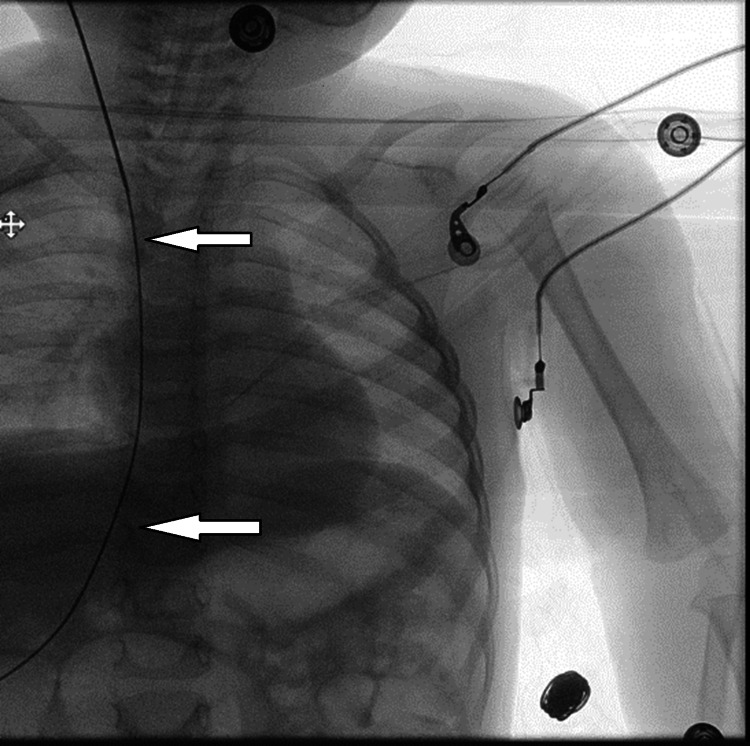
An anteroposterior chest X-ray, showing the guidewire of the catheter (white arrows) advancing into the inferior vena cava (IVC) via the right internal jugular vein, confirming its placement.
